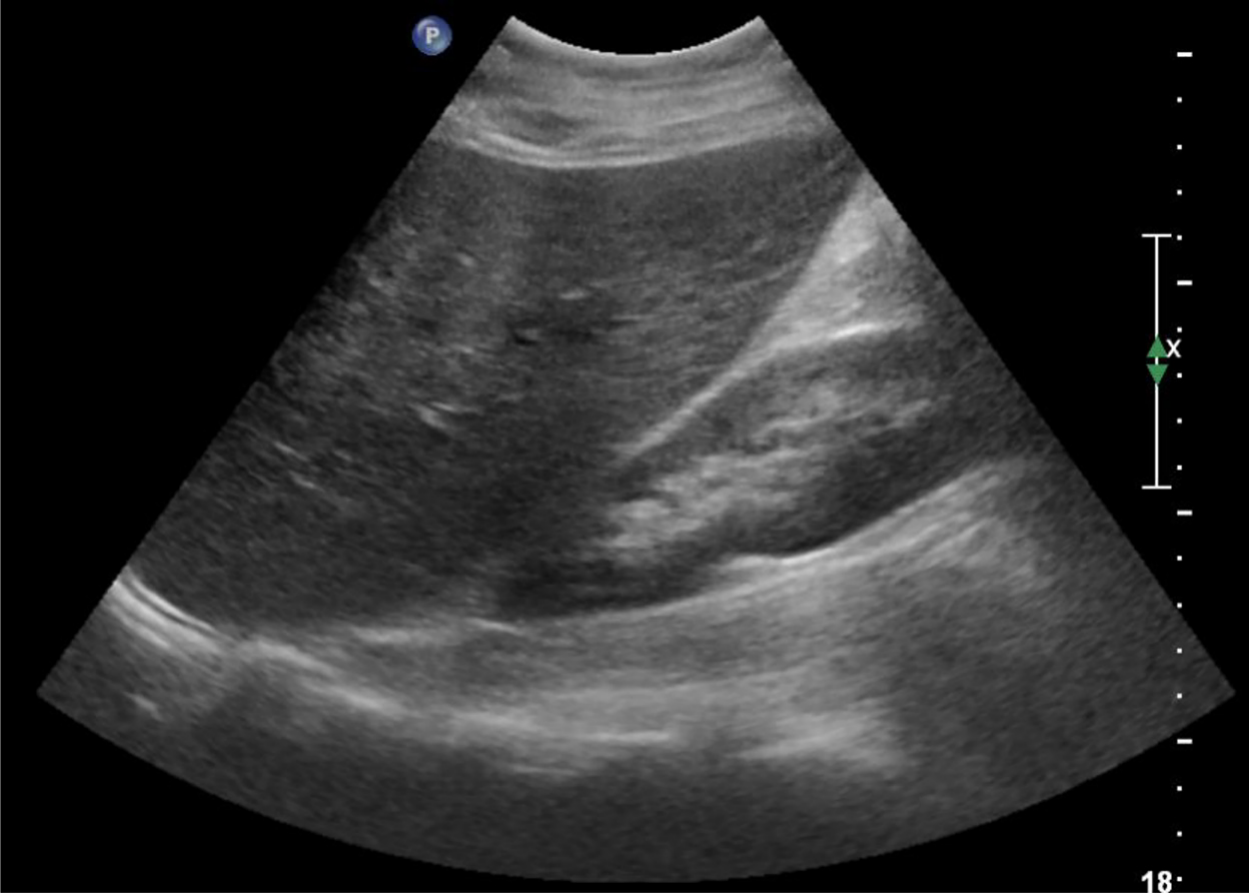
Right upper quadrant sonogram showing hepatomegaly.
